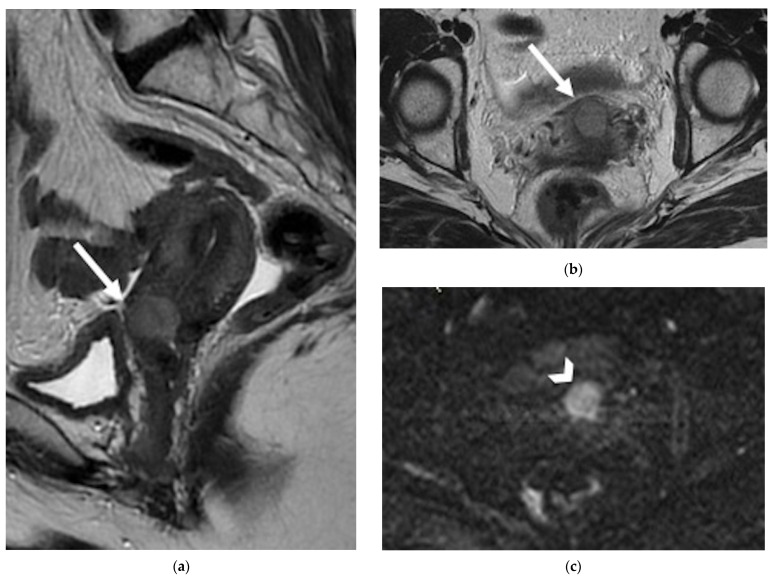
A 42-year-old patient with history of cervical cancer treated with chemoradiation therapy. (a) Sagittal and (b) axial FSE T2-weighted images show a nodular hyperintense lesion in the anterior aspect of the cervix, causing interruption of the hypointense cervical stroma, consistent with recurrence (arrows). (c) Axial diffusion-weighted image demonstrates restricted diffusion of the lesion (arrowhead).
